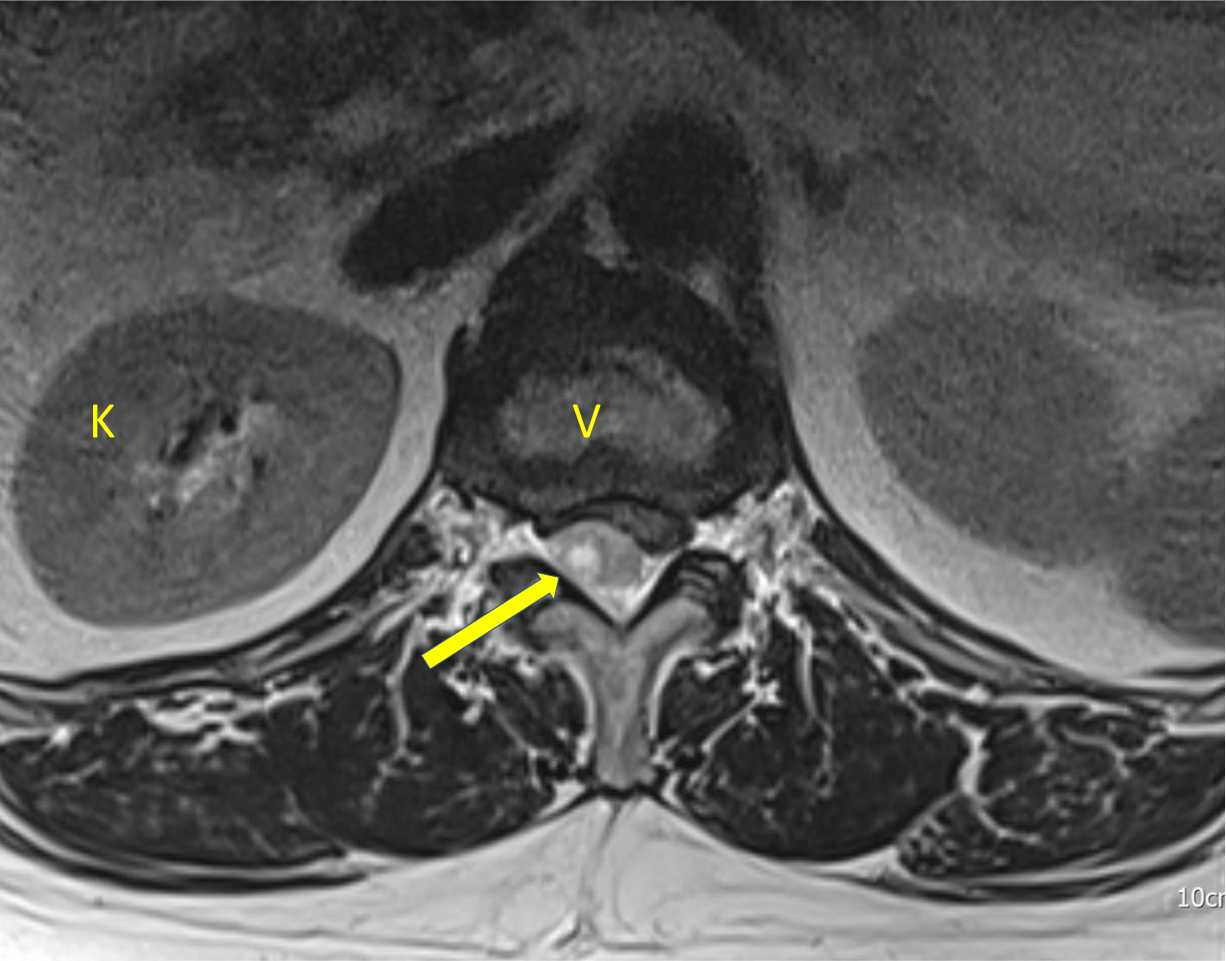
Lumbosacral magnetic resonance imaging without contrast, axial view. V: vertebrae, K: kidney, arrow: epidural hematoma occupies the spinal canal and compresses the spinal cord
